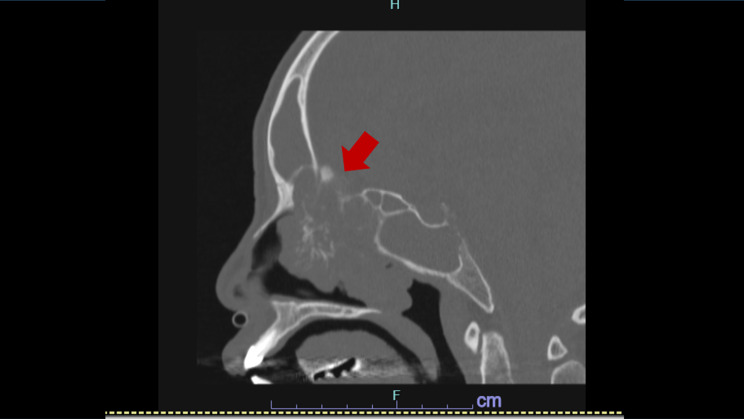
Computed tomography (CT) of the paranasal sinuses. Sagittal CT images demonstrate opacification of the left paranasal sinuses with a mass lesion consistent with a sinonasal papilloma. Partial bone destruction of the sinus wall is observed (arrow), suggesting locally aggressive disease. These findings are consistent with complicated sinusitis with a potential risk of extension beyond the sinus cavity
